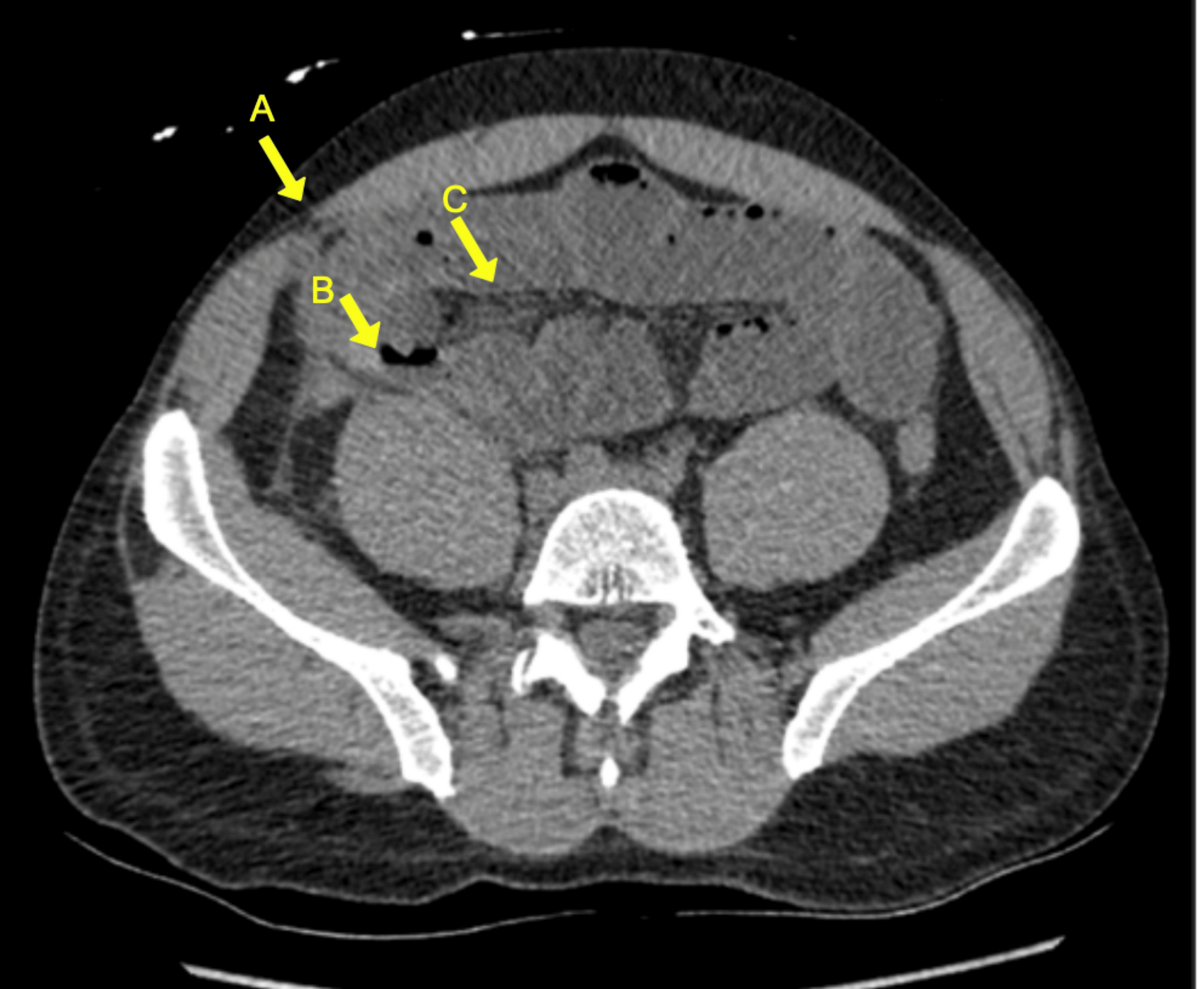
Abdominal computed tomography (CT) scan. Axial non-contrast CT of the abdomen demonstrates pneumoperitoneum (A), dilated fluid-filled small bowel loops (B), and mesenteric stranding (C). These findings are concerning for bowel ischemia with perforation, later confirmed intraoperatively.
